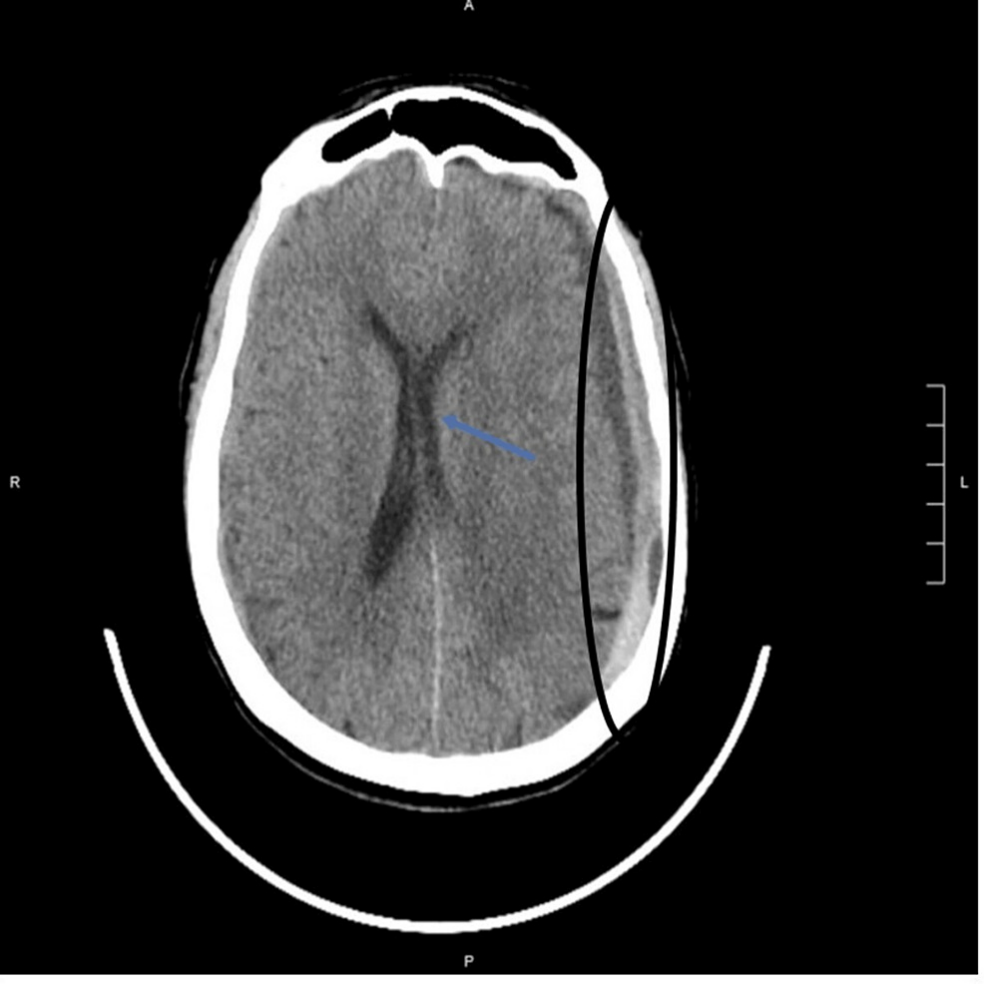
CT Head Extensive subdural hemorrhage is identified along the left cerebral convexity, measuring up to 11 mm in maximal transverse thickness (circle).  There is a 7 mm rightward shift of the midline (arrow).
